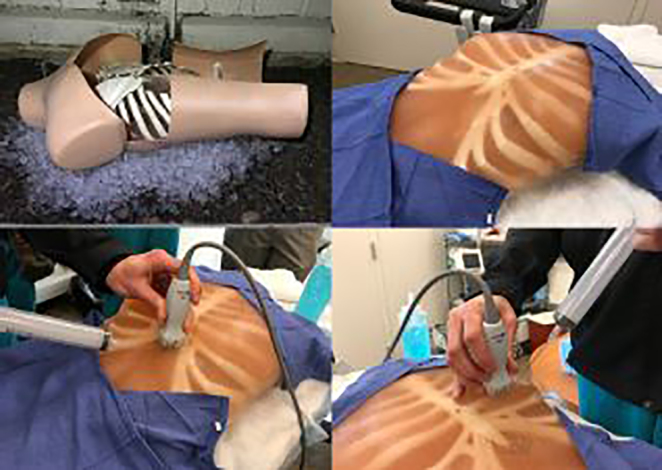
Modified ultrasound-guided pericardiocentesis trainer using a plastic dress form torso turned upside down to act as a mold.
